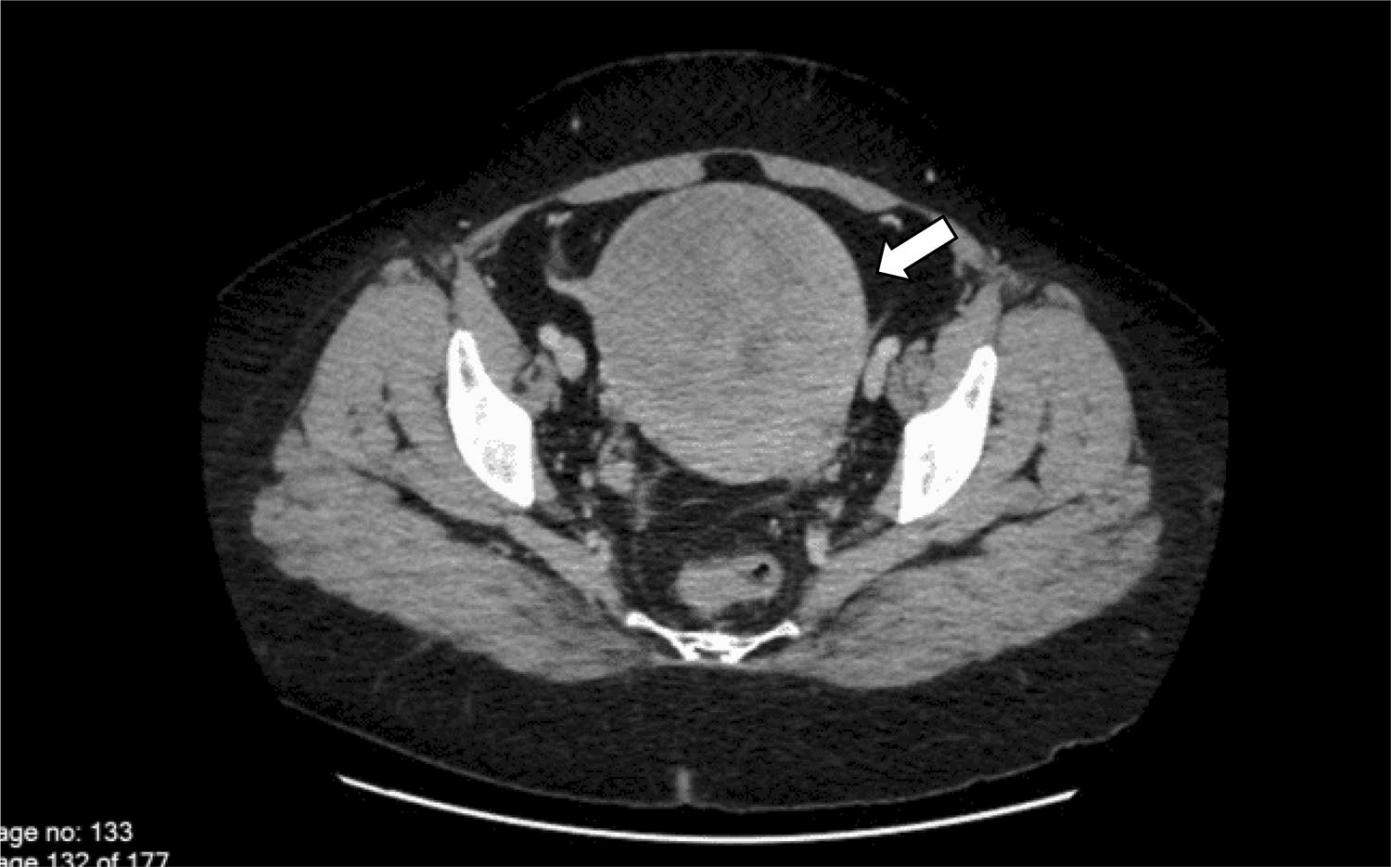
Pelvic CT examination revealing a well-defined 11 cm pelvic mass without further abnormalities
